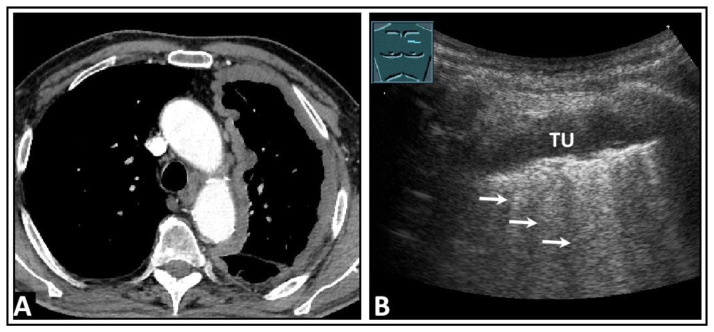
A patient with dyspnea, thoracic pain, and confirmed left-sided pleural mesothelioma on computed tomography (A). (B) On lung ultrasound, there is a widespread pleural consolidation (TU) on the left side, with multiple B-lines (arrows) and a rough lung surface adhering to the tumor layer.
